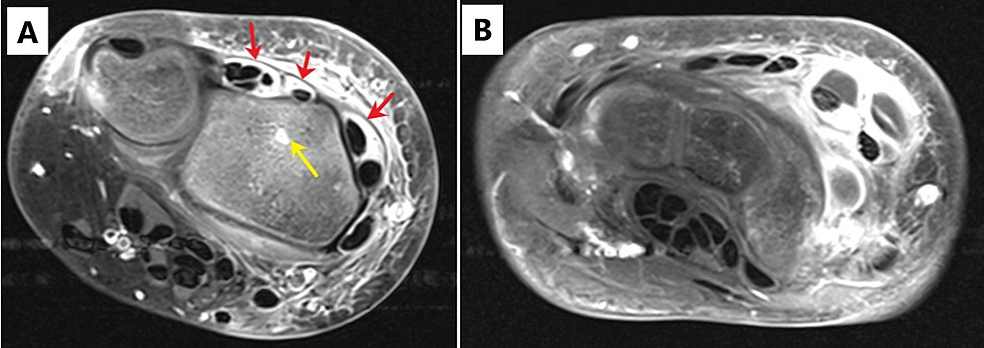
Magnetic resonance imaging (MRI) of the right wrist. (A and B) Axial T1-weighted fat saturation, postgadolinium contrast demonstrating fluid in multiple extensor tendon sheaths (red arrows) with surrounding edema, suggestive of pyogenic extensor tenosynovitis. There was also enhancement within the distal radius, suggestive of possible osteomyelitis (yellow arrow).
